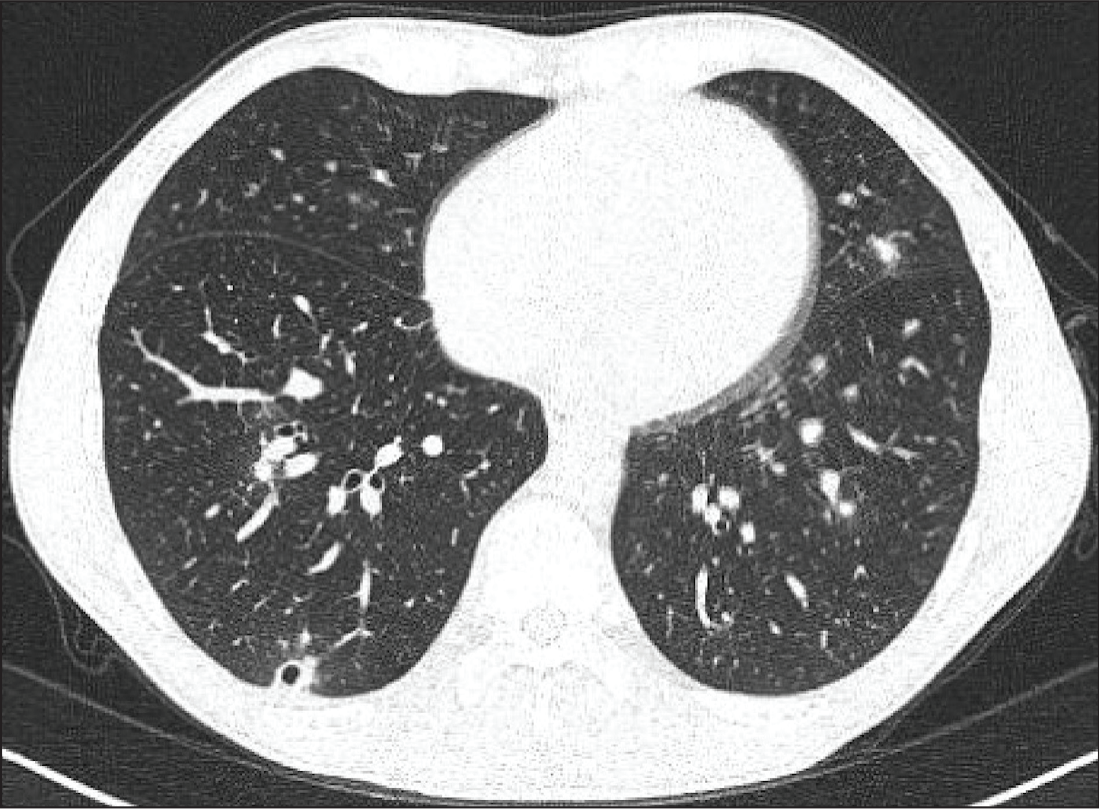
Computed tomography of chest of Case 1, histoplasmosis in Canadian traveller, 2025
